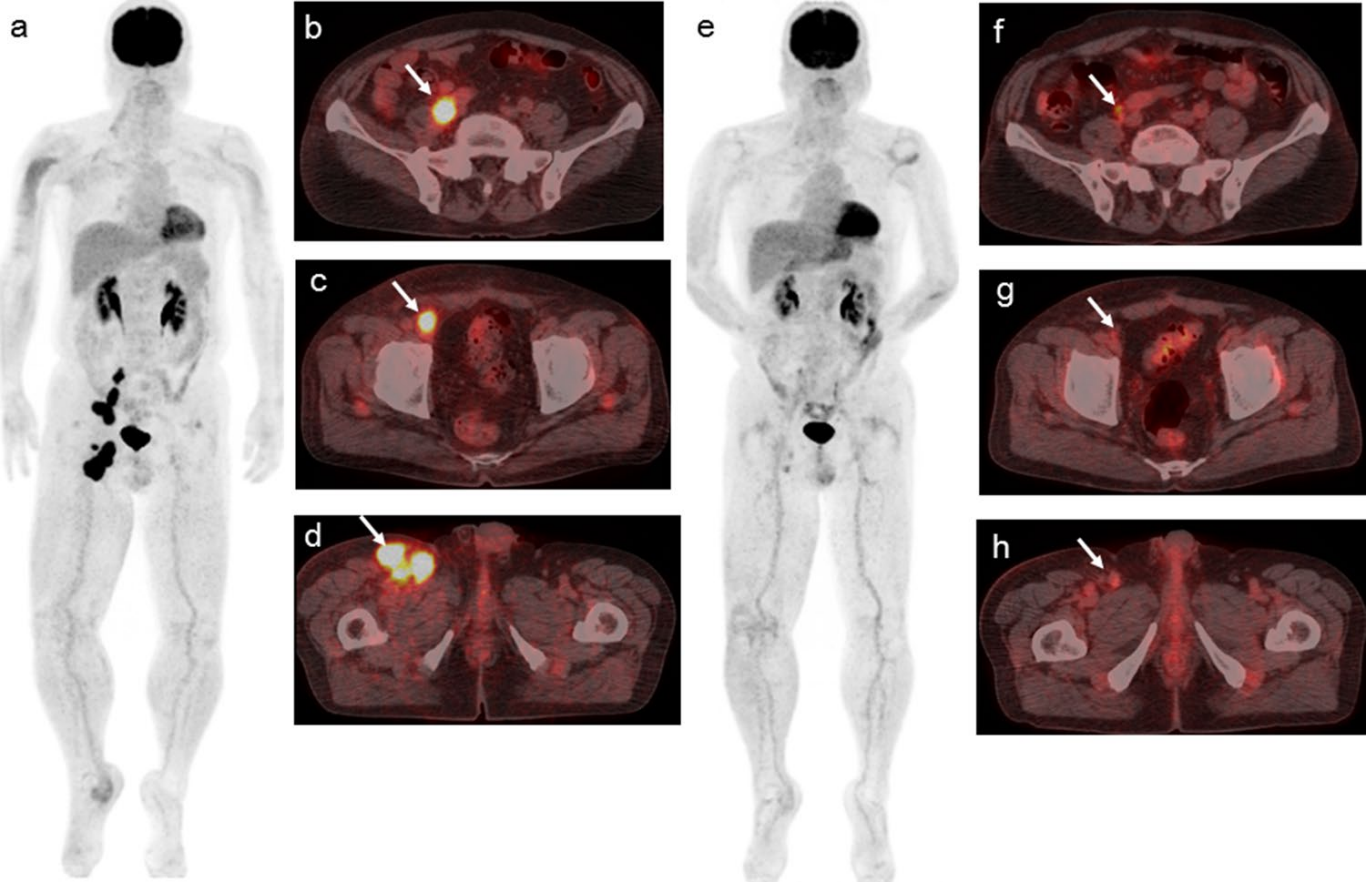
A 66-year-old man with postoperative recurrence malignant melanoma with right pelvic and inguinal nodal metastases received nivolumab. Baseline ^18^F-FDG PET/CT [maximum intensity projection (MIP) (a) and fused transaxial (b–d) images] shows abnormal ^18^F-FDG uptake in the right (b) common iliac node metastasis (arrow), c external iliac node metastasis (arrow) and d inguinal node metastases (arrow). The follow-up ^18^F-FDG PET/CT after nine courses of nivolumab therapy [MIP (e) and fused transaxial (f–h) images] show almost disappearance of ^18^F-FDG uptake in these nodal metastases (arrows). Because post FDG-uptake of these nodal metastases was slightly higher than the surrounding tissue and the reductions of the sum of harmonized SUV_max_ were 83.3% (from 43.95 to 7.33), the status was PMR according to harmonized EORTC criteria. Because post ^18^F-FDG uptake of all three nodal metastases was less than the liver activity, the response status according to harmonized PERCIST and harmonized imPERCIST was CMR. The patient was alive without progression 68.1 months after the initiation of nivolumab. Pretreatment harmonized SUV_max_/SUV_mean_/SUL_peak_ of the right common iliac, external iliac, and inguinal nodal metastases were 14.67/9.32/9.6, 13.09/9.21/9.37, and 16.19/10.09/11.55, respectively. Pretreatment harmonized whole-body MTV and TLG were 72.46 and 714.51, respectively. Posttreatment harmonized SUV_max_/SUV_mean_/SUL_peak_ of the right common iliac, external iliac, and inguinal nodal metastases were 3.26/1.65/1.96, 1.97/1.43/1.62, and 2.13/1.51/1.67, respectively. Posttreatment liver SUL (mean + 2 standard deviations) was 2.33. Posttreatment harmonized whole-body MTV and TLG were 19.35 and 30.58, respectively
